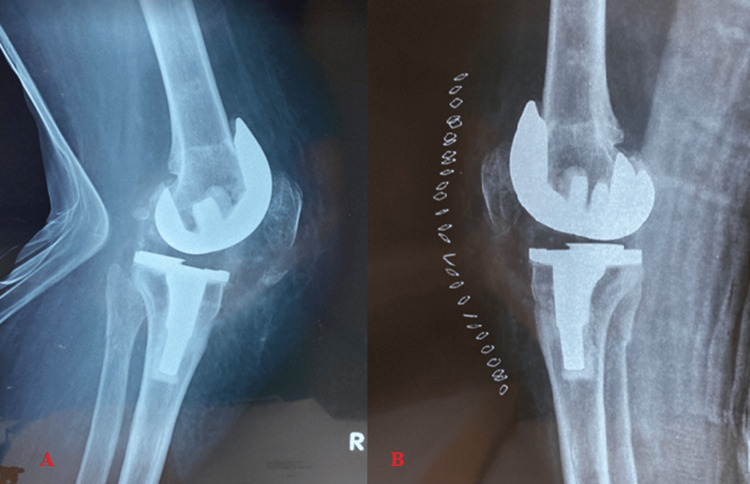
Non-traumatic patella periprosthetic fracture in a 65-year-old female patient of our clinic, identified during the 2-year routine follow-up. The patient claimed severe anterior knee pain and loss of extension. A) Detection of the fracture of the distal pole of the patella in lateral knee X-ray. B) Immediate postoperative lateral knee X-ray after internal fixation of the fracture using a 1.5 mm bone tunnel with bone sutures. Written informed consent was signed by the patient to publish the X-rays.
